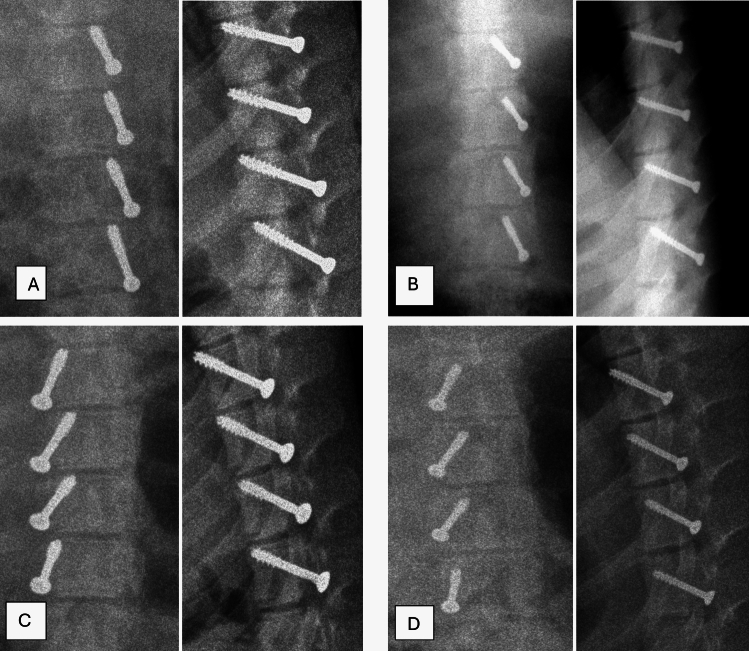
A-D Anteroposterior and lateral radiographs demonstrating spinal epiphysiodesis trajectory (SET) screw placement at levels T9 to T12. Case 1: A immediately postoperatively and B at 3-month follow-up. Case 2: C immediately postoperatively and D at 3-month follow-up
